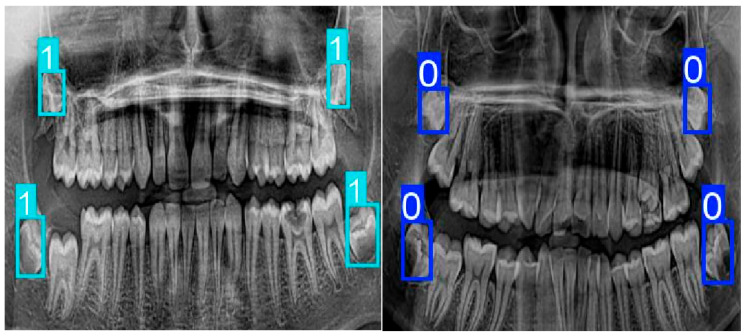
Bounding-Box based visualization of sex prediction results on panoramic dental radiographs (1 = Male, 0 = Female).
